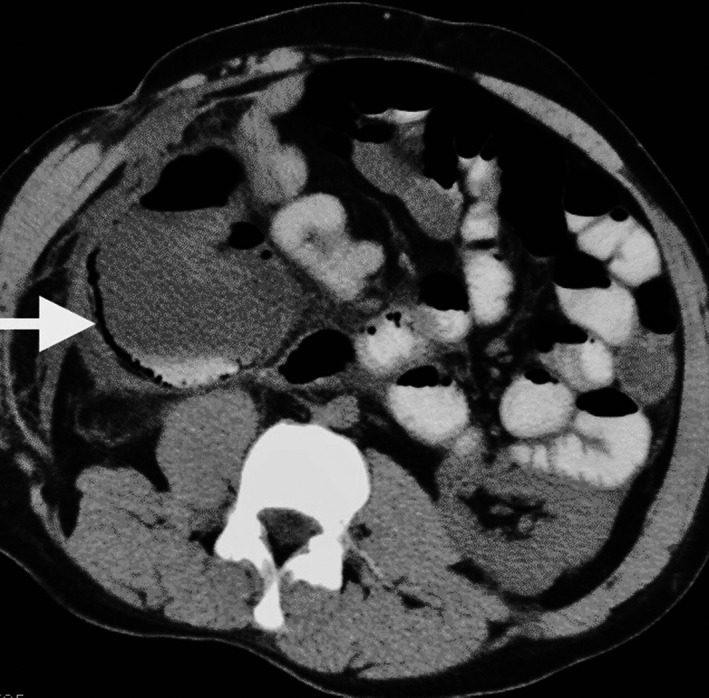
CT abdomen and pelvis showing pneumatosis intestinalis (arrow)
